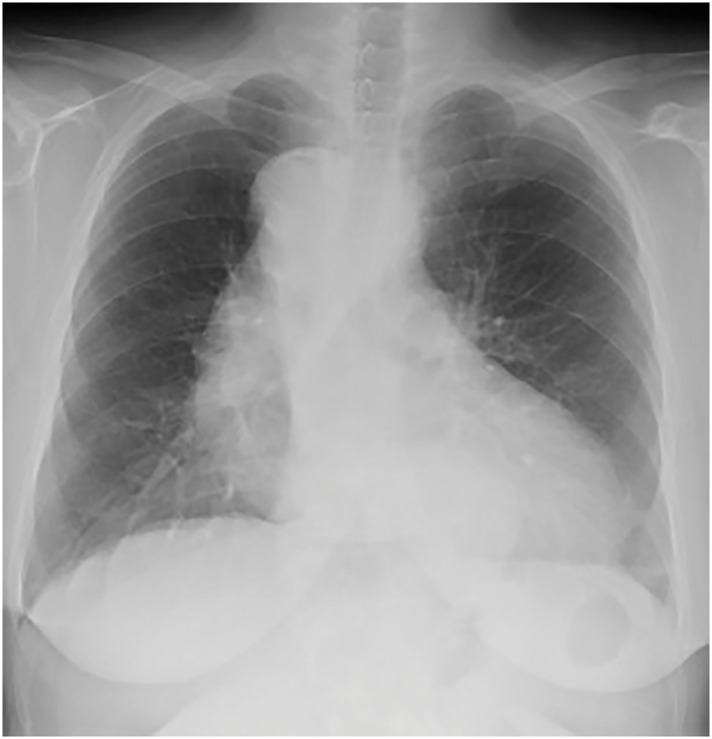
Chest X-ray showing an RAA. RAA, right aortic arch
